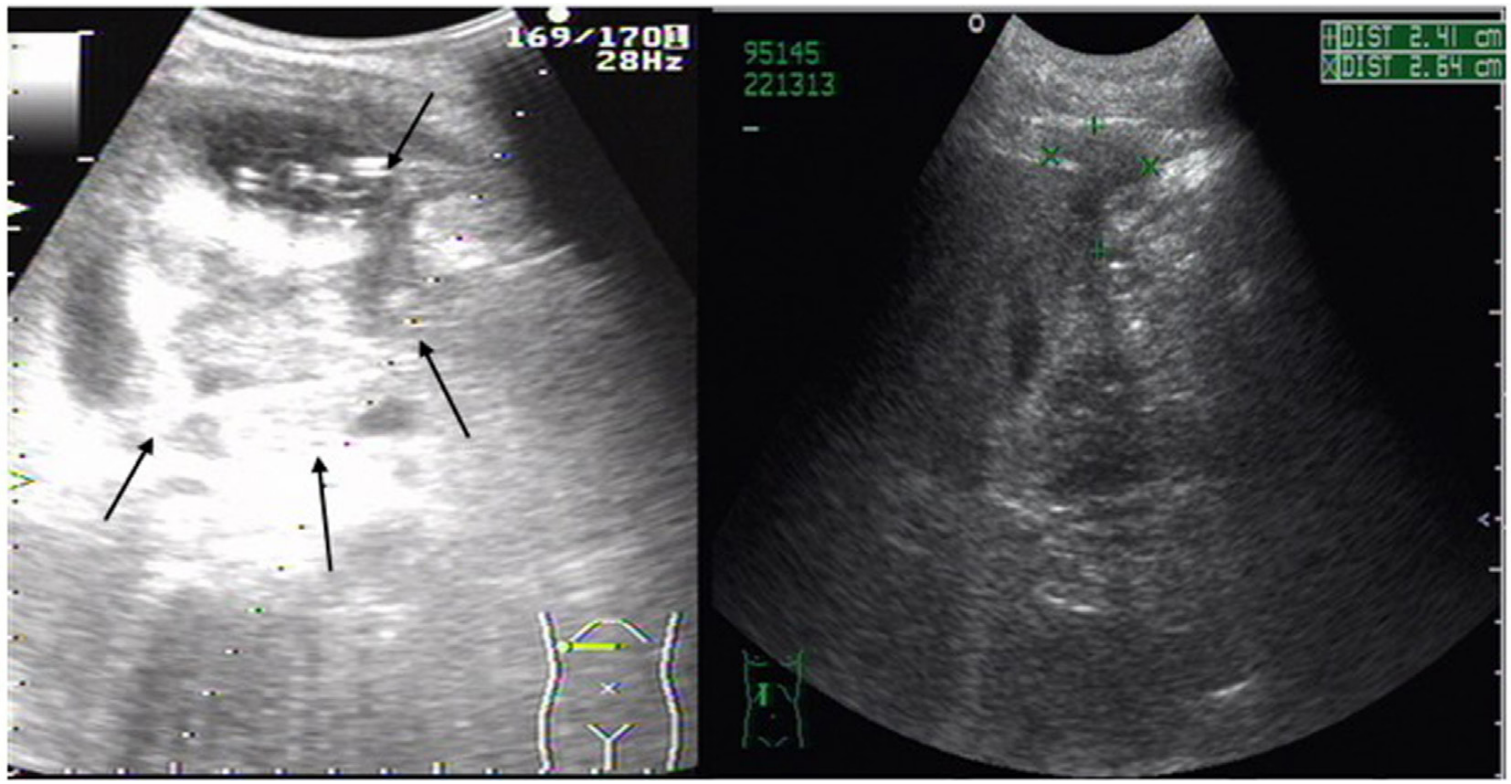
Abdominal ultrasonography scan of liver abscess in right lobe of the liver. Large (82 mm × 78 mm) multiple low-signal areas (shown by arrows) were detected before percutaneous abscess drainage. Its size (+, ×) was greatly reduced after percutaneous liver abscess drainage.
